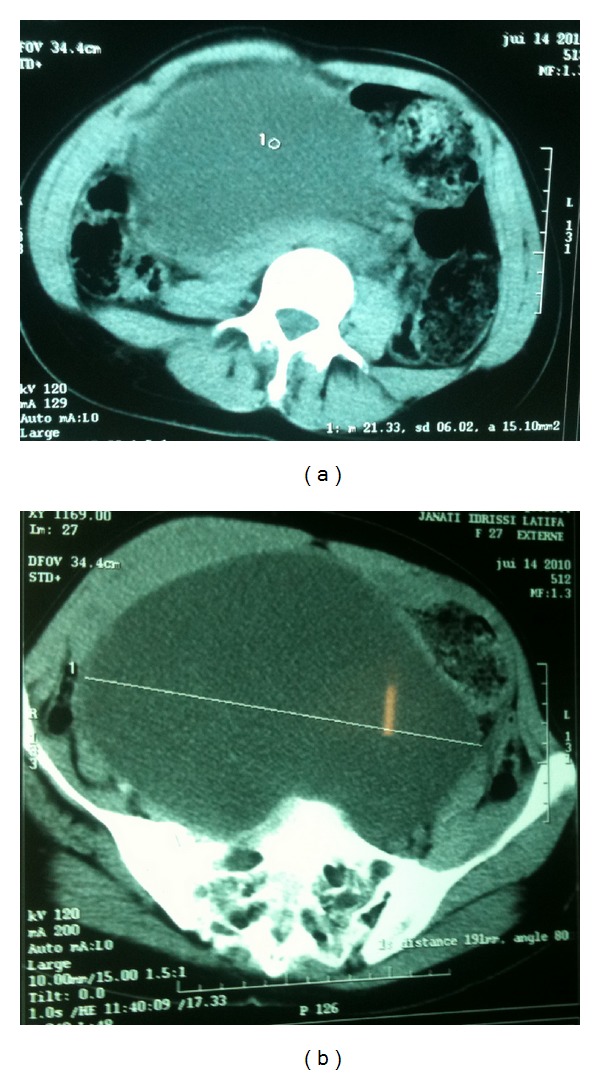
Computed tomography showed a wellcircumscribed mass displacing the uterus forward with left ureterohydronephrosis respecting the cortex suggesting a giant ovarian cyst.
